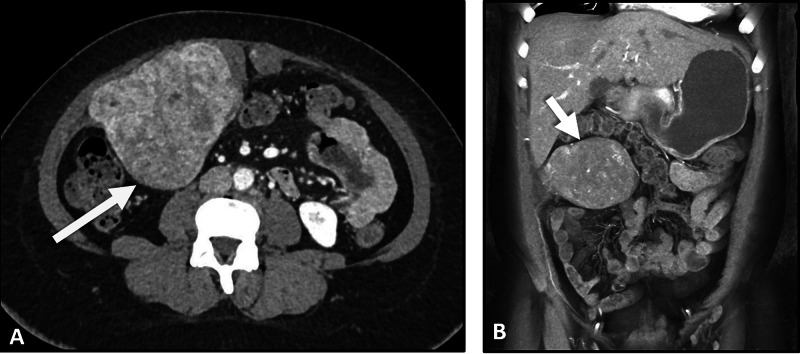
Axial (A) and coronal (B) abdominal CT images demonstrating a heterogenous left-sided abdominal mass lesion (arrow) with enhancement originating from the underlying muscular structures. CT, computed tomography
